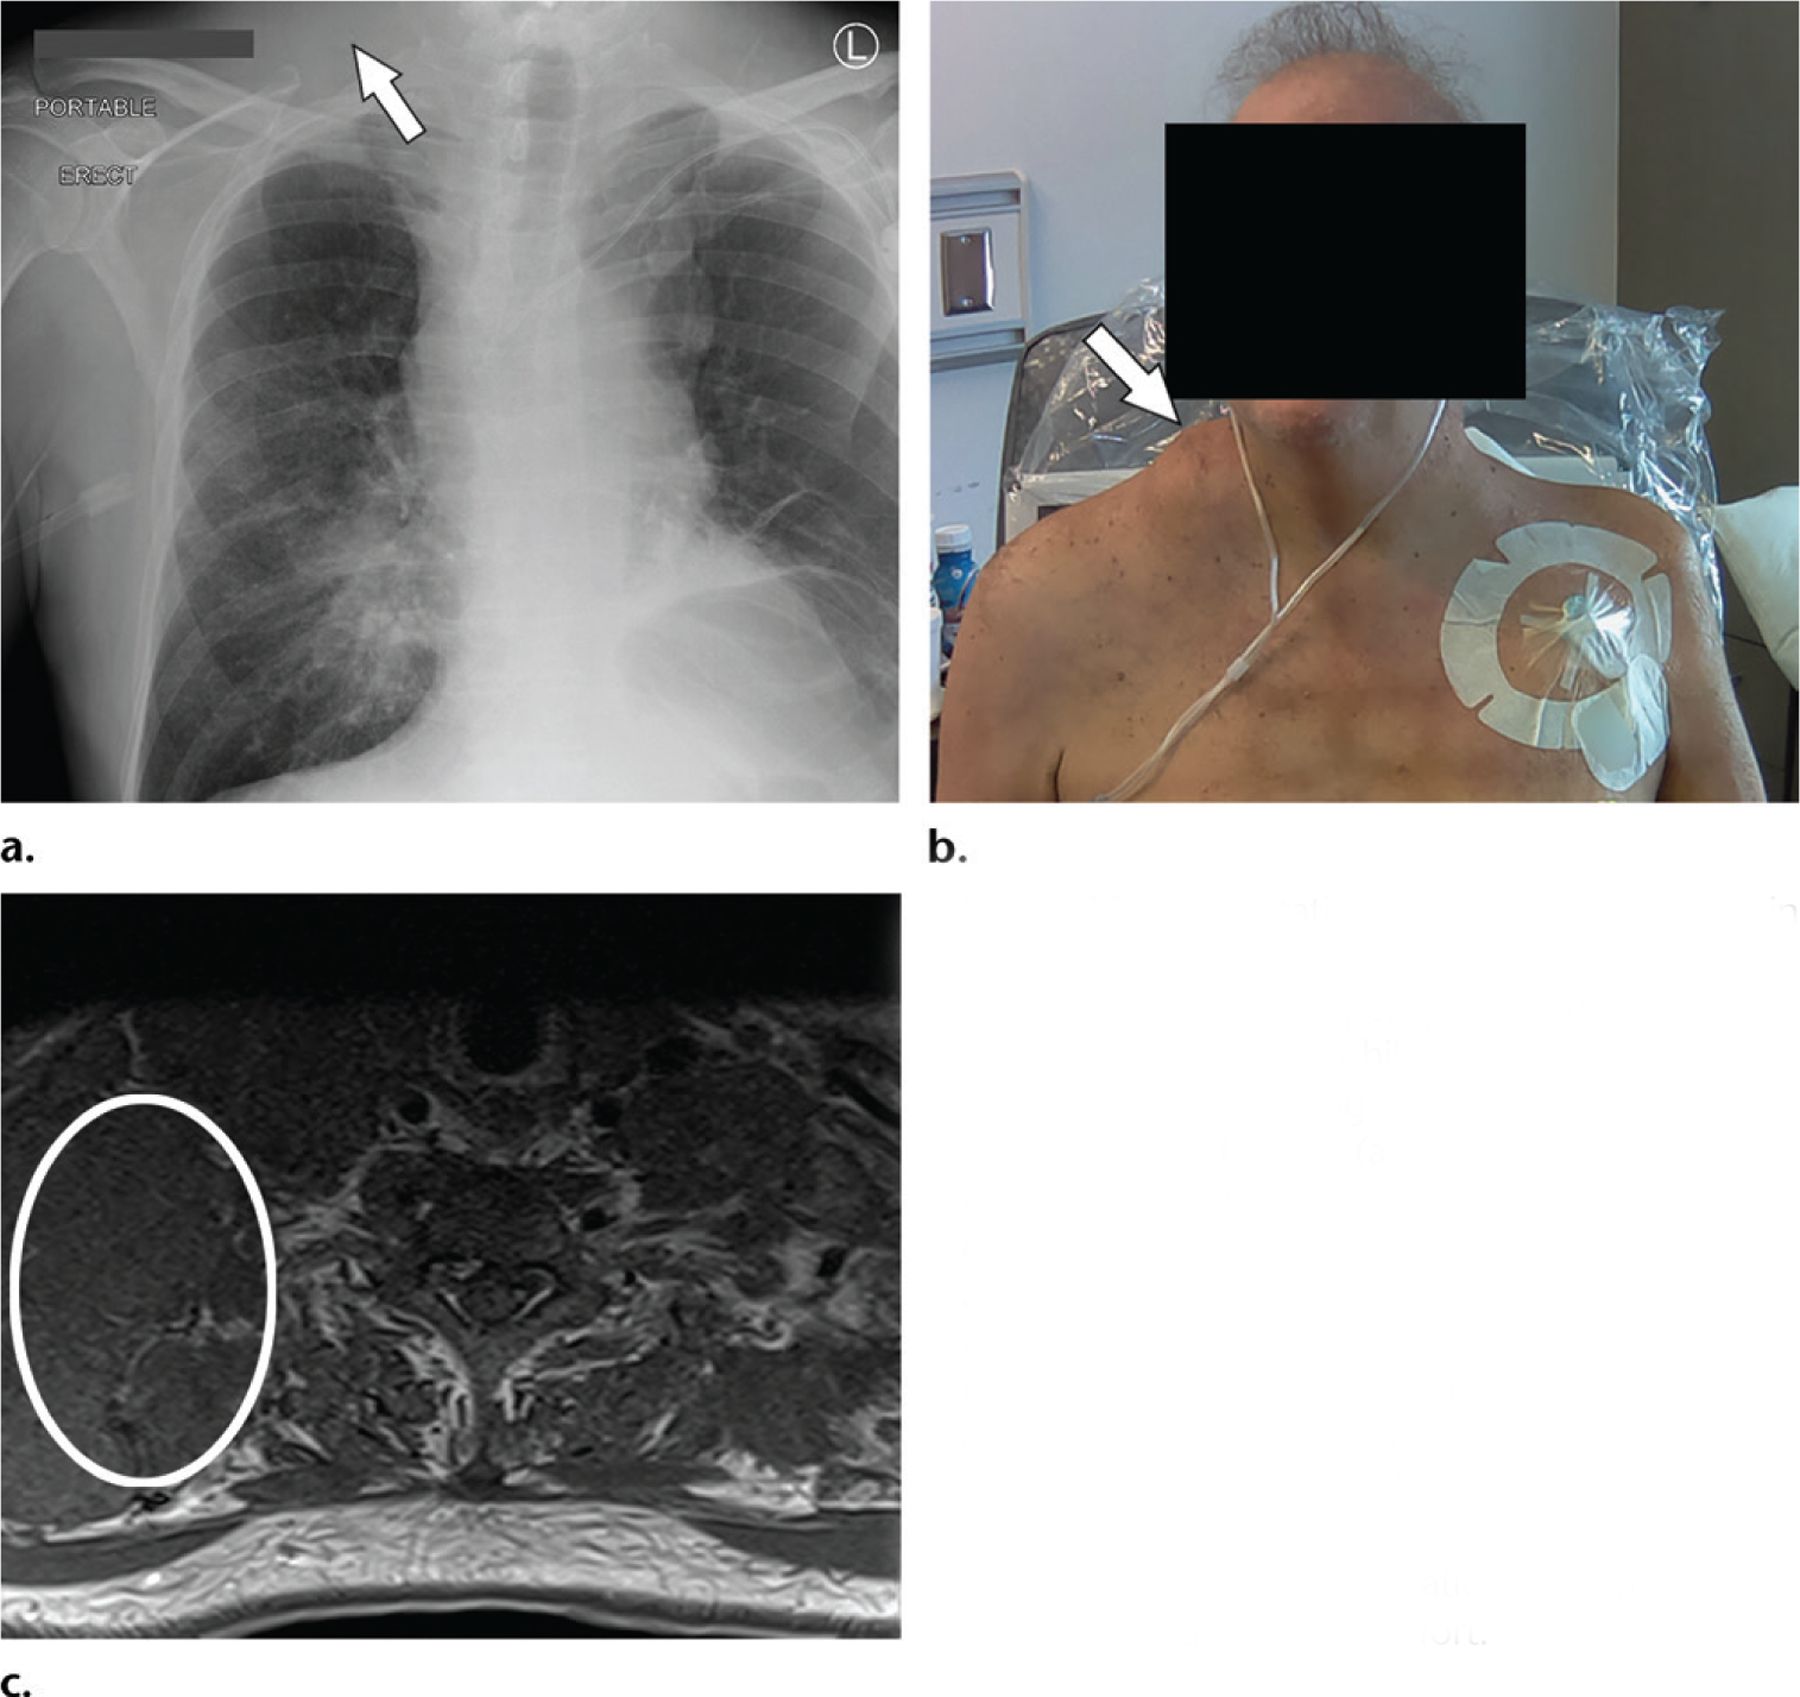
Metastatic spread of primary cancer in a man who underwent portable chest radiography for evaluation of chest port placement. (a) Frontal radiograph shows right hilar fullness and elevation of the left hemidiaphragm. (b) Photograph shows asymmetric soft tissue (arrow in a and b) in the right suprascapular region, which is not as obvious on the radiograph. (c) Axial MR image shows a right neck mass (circle). Although information about the right neck mass was available on the MR image obtained prior to the radiograph, the radiologist interpreting the portable radiograph would not be likely to open the MRI study. The findings on the photograph could prompt the radiologist to report a possible metastatic nodal mass in the right neck without much effort.
